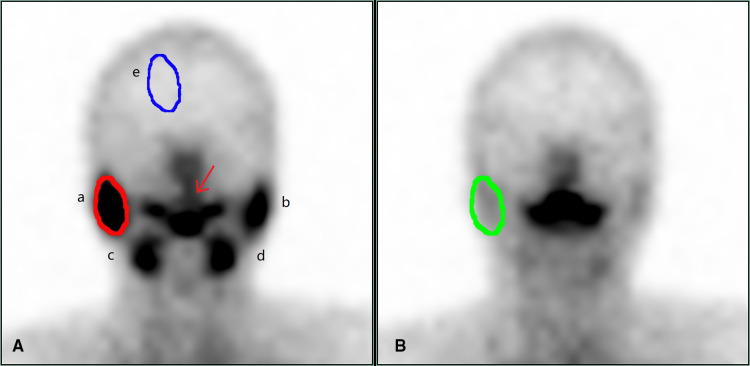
Dynamic salivary gland scintigraphy. (A) is the frame with the highest radioactive count uptake by the salivary glands, and (B) is the frame with the lowest radioactive counts after the salivary gland secretion. In (A), a is the right parotid gland, b is the left parotid gland, c is the right submandibular gland, d is the left submandibular gland, and e is the background, draw the ROI to obtain the radioactivity count, which is (B) in the formula. The red arrow is the saliva secreted by the salivary glands. In (A), the red circle outlines the right parotid gland, and the ROI is drawn to obtain the radioactive count, which is the highest count concentrated by the right parotid gland, which is the C_max_ in the formula. In (B), the green circle outlines the right parotid gland, and the ROI is drawn to obtain the radioactive count, which is the lowest count after the secretion by right parotid gland, that is C_min_ in the formula. Draw b, c, d in turn, and calculate the concentrate index and secretion index of each gland according to the following formulas. Concentrate index (CI) = (C_max_–B)/ B secretion index (SI) = (C_max_–C_min_)/C_max_ × 100%. C_max_ = gland highest radioactive count; C_min_ = the lowest gland radioactivity count after vitamin C stimulation; B = background radioactivity count.
